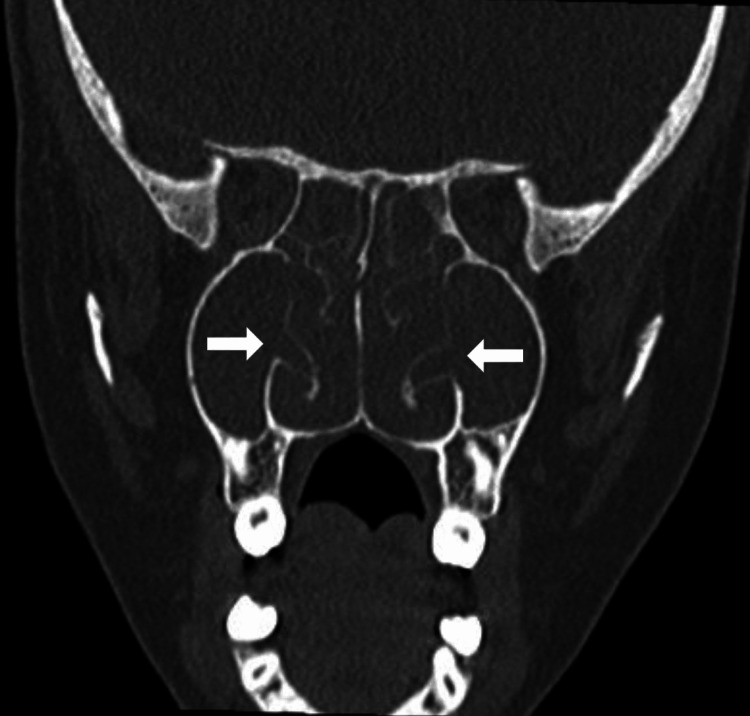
Non-contrasted paranasal sinuses CT scan, coronal view for case 2. There is a complete opacification of sino-nasal cavities on both sides due to nasal polyps with opacified lamellar pneumatization of the inferior turbinate bilaterally. The pneumatization is communicating with the maxillary sinus bilaterally (arrows).
